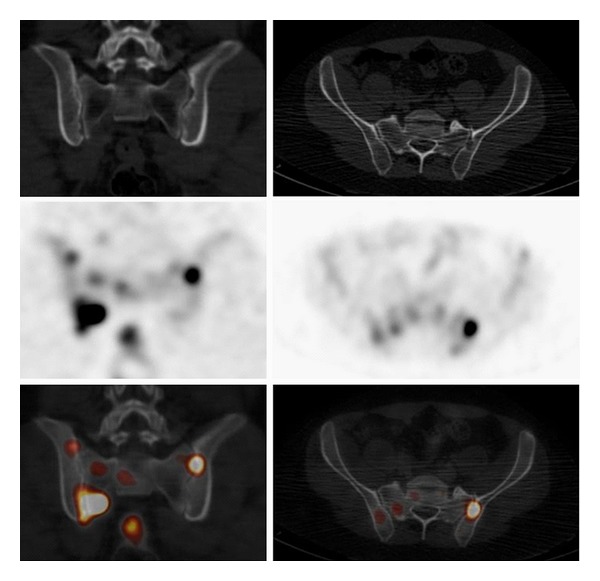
The figure shows sacroiliac joint involvement as hypermetabolic lesions as seen on ^18^F-FDG-PET/CT. Left row: coronal CT, PET, and fused PET/CT slices. Right row: transverse CT, PET, and fused PET/CT slices.
